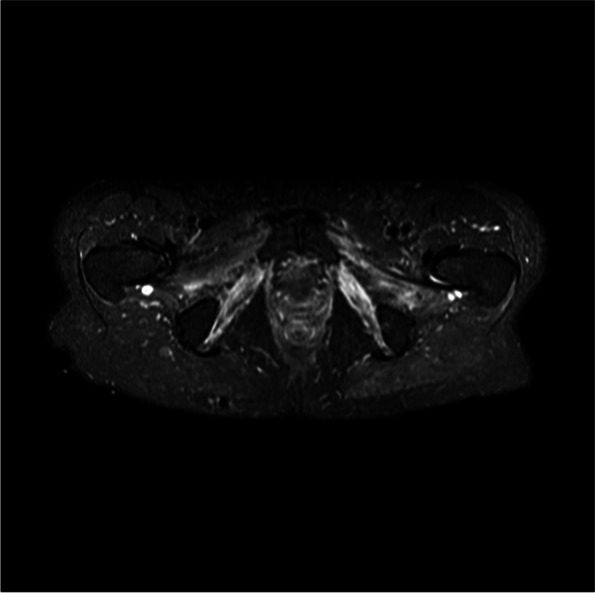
MRI examination of the pelvis (the axial STIR image) 42 years after radiotherapy demonstrates a high signal within the pelvic muscles bilaterally, indicating an acute-to-subacute phase of muscle denervation
